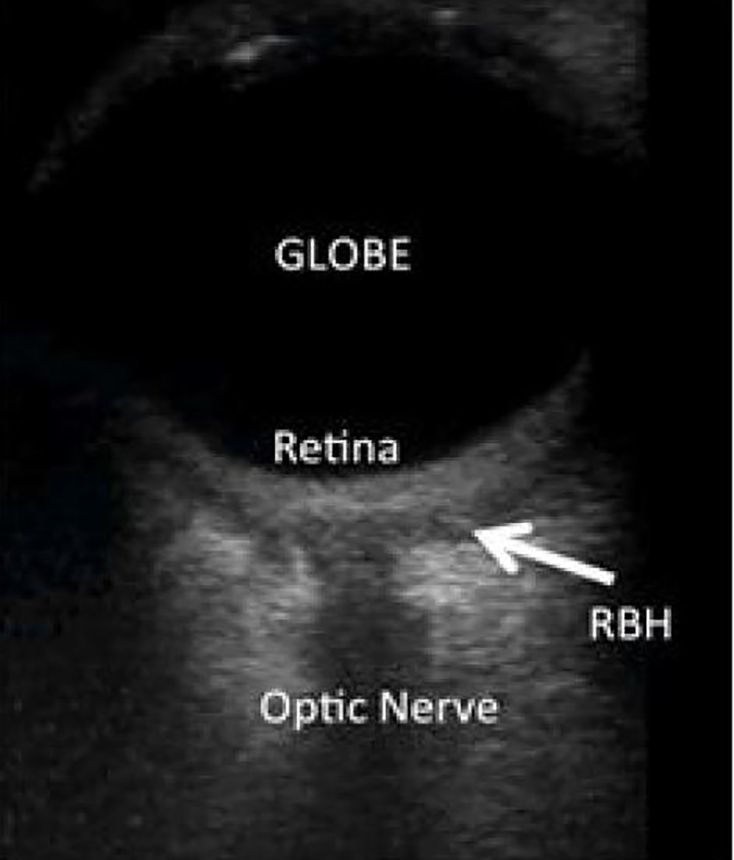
Point-of-care ultrasound, transverse view, of the left orbit using a linear probe demonstrating a RBH (arrow). *RBH*, retrobulbar hematoma.
